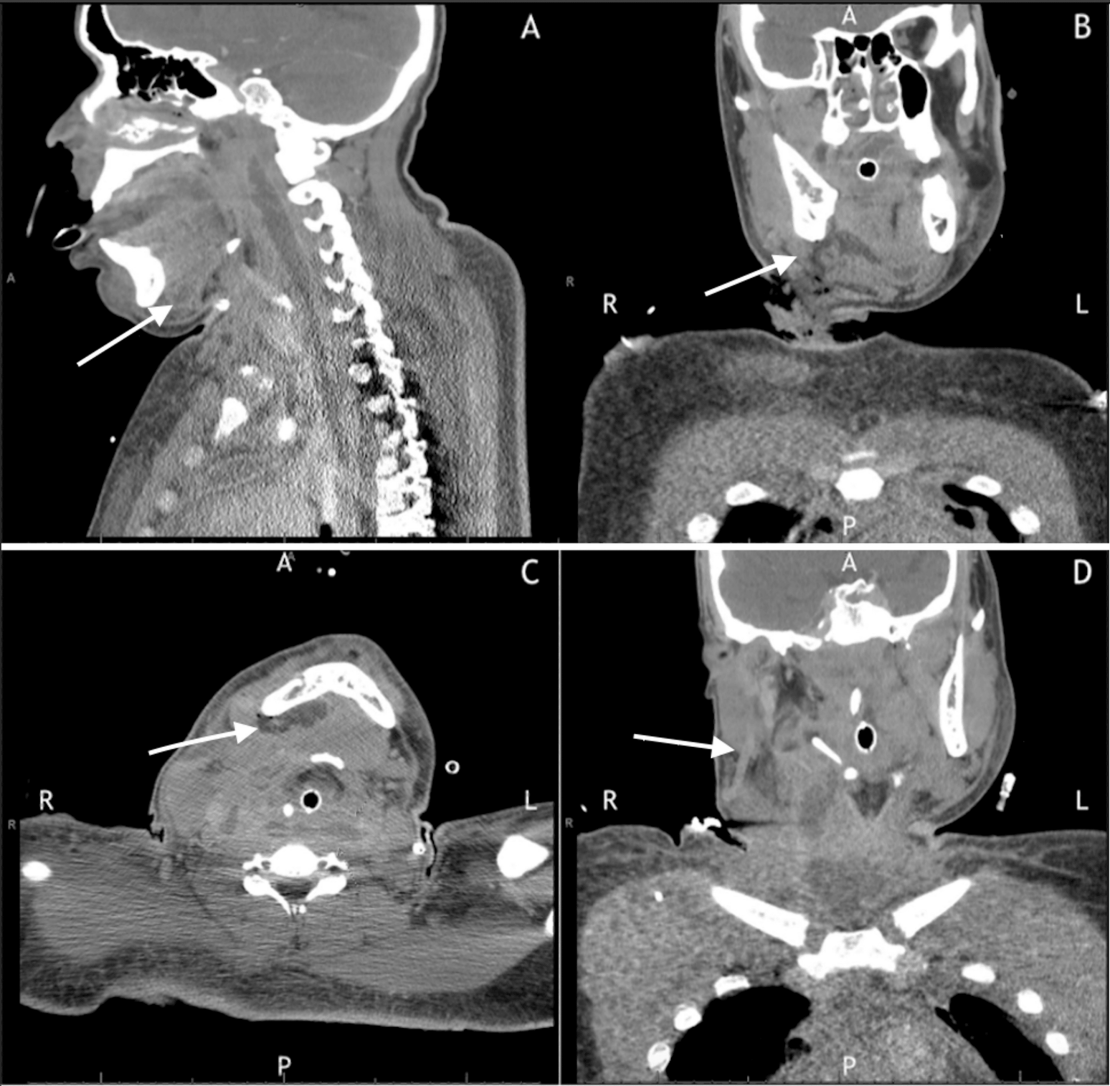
CT scan after surgical intervention CT: Computed tomography Evolution to mediastinal abscess on follow-up imaging. (A) Sagittal contrast-enhanced CT neck demonstrating organized fluid collections with enhancing walls in the mediastinum, representing abscess formation. (B) Coronal view showing residual cervical emphysema with new rim-enhancing collections along the surgical drainage pathways. Note the developing pleural reaction and residual pneumomediastinum. (C) Axial view further demonstrating fluid collection representing abscess formation. (D) Coronal view showing the posterior extent of the abscess near the mandible
